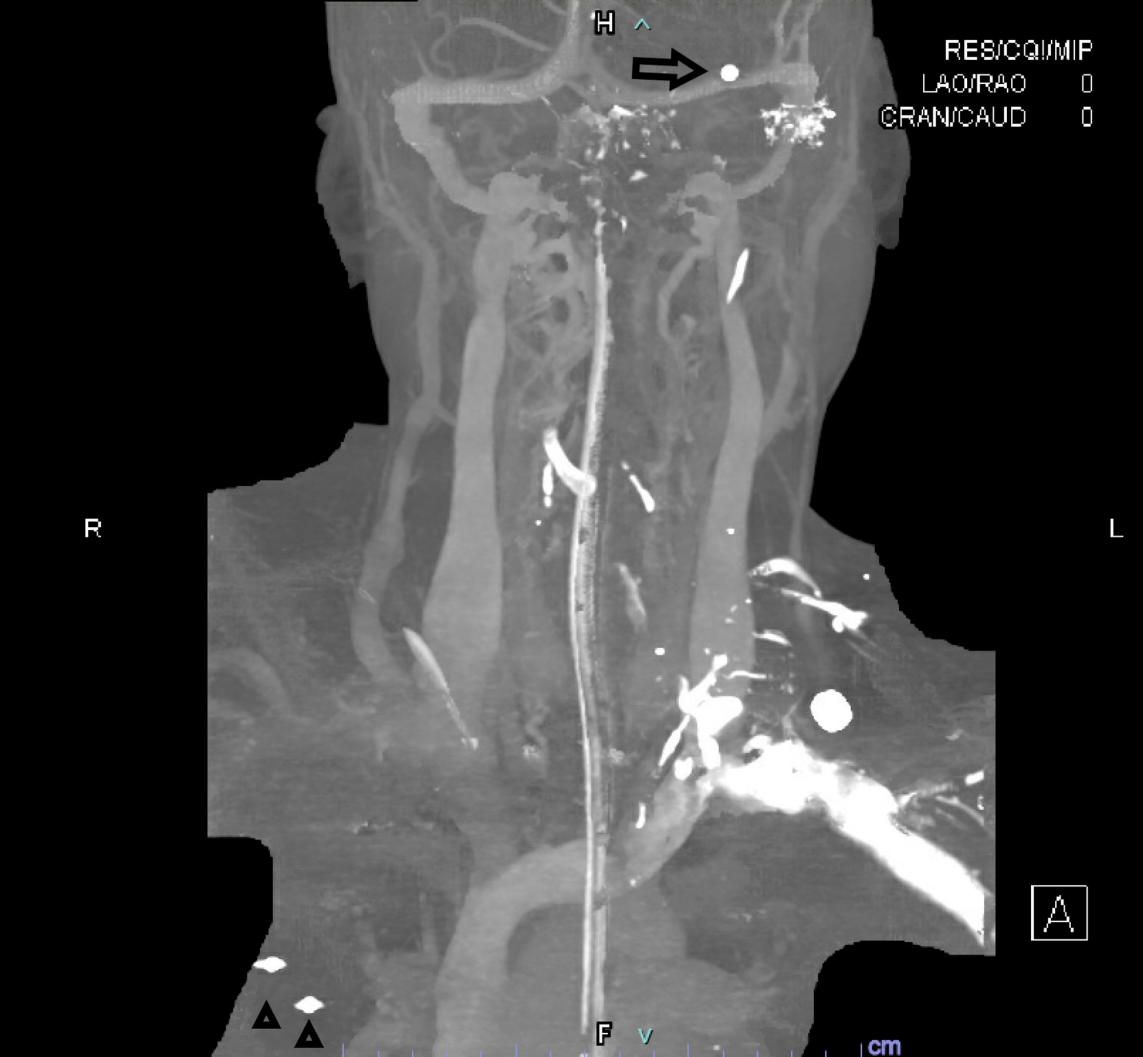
CTA head and neck coronal MIP Arrow=Pellet in location of left MCA Arrowheads=Pellets in the right upper lobe
